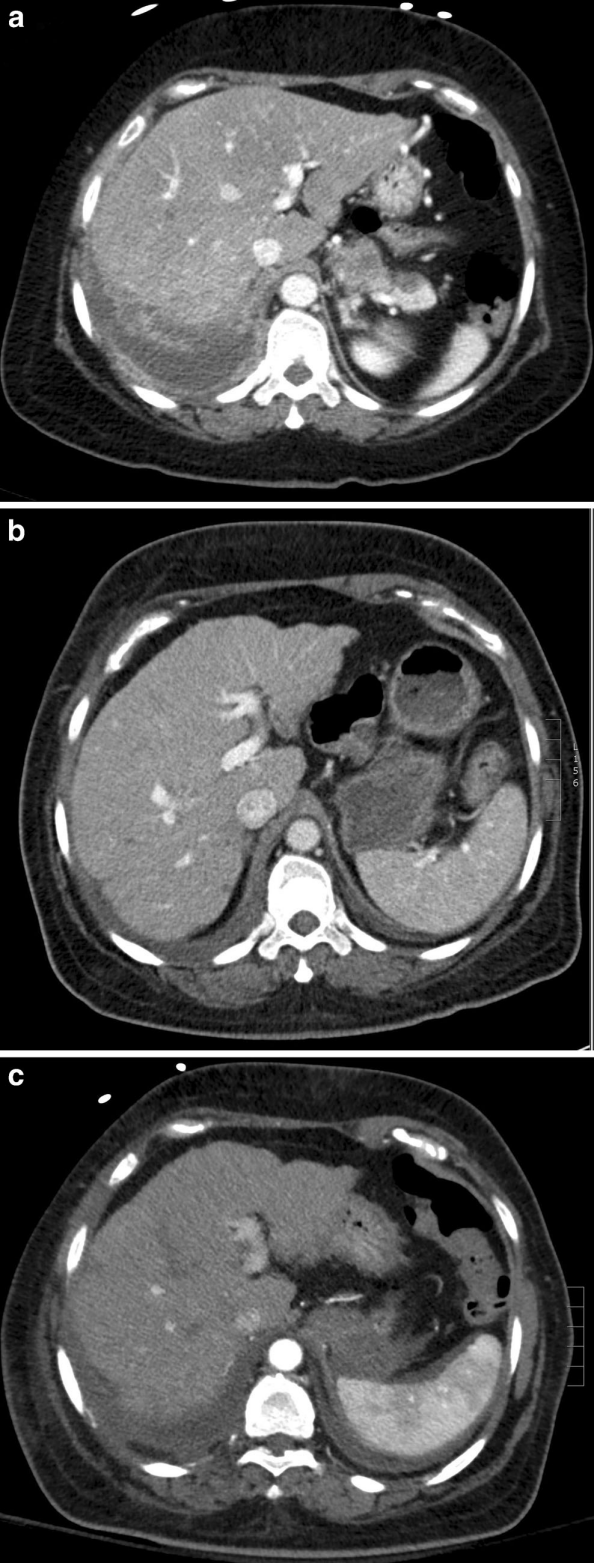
A 48-year-old female with metastatic breast cancer status post treatment with docetaxel and epirubicin. a Axial contrast-enhanced CT before chemotherapy shows normal-appearing liver with a smooth surface. b Repeat CT 6 months after initiation of treatment shows nodularity of the left hepatic lobe. c Repeat CT 7 months after initiation of treatment shows diffuse nodularity and capsular retraction consistent with pseudocirrhosis
